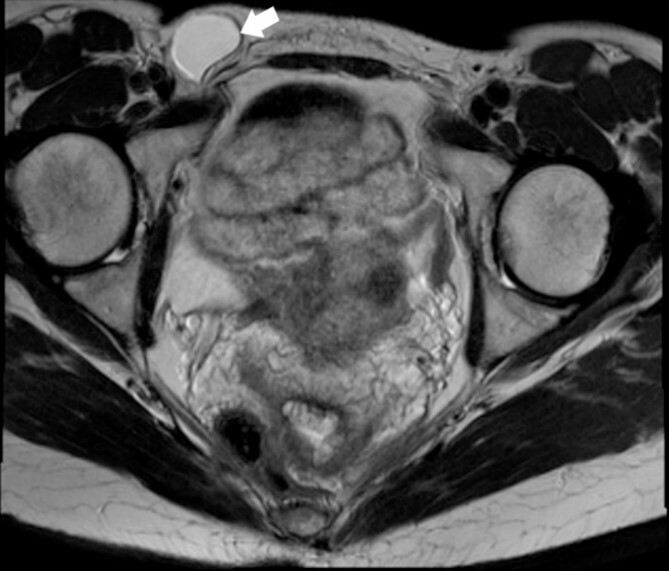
MRI of the Cyst of the Canal of Nuck–T1 sequence. This image highlights the cyst (indicated by the white arrow) within the inguinal region.
